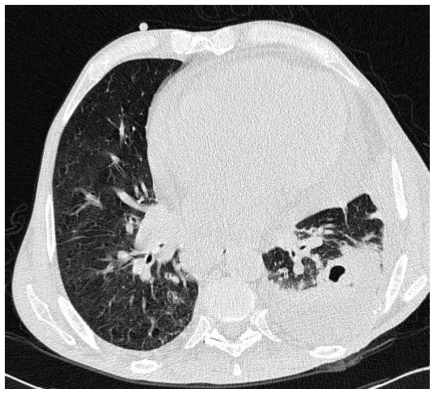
Patient’s lung nodule at CT scan.
